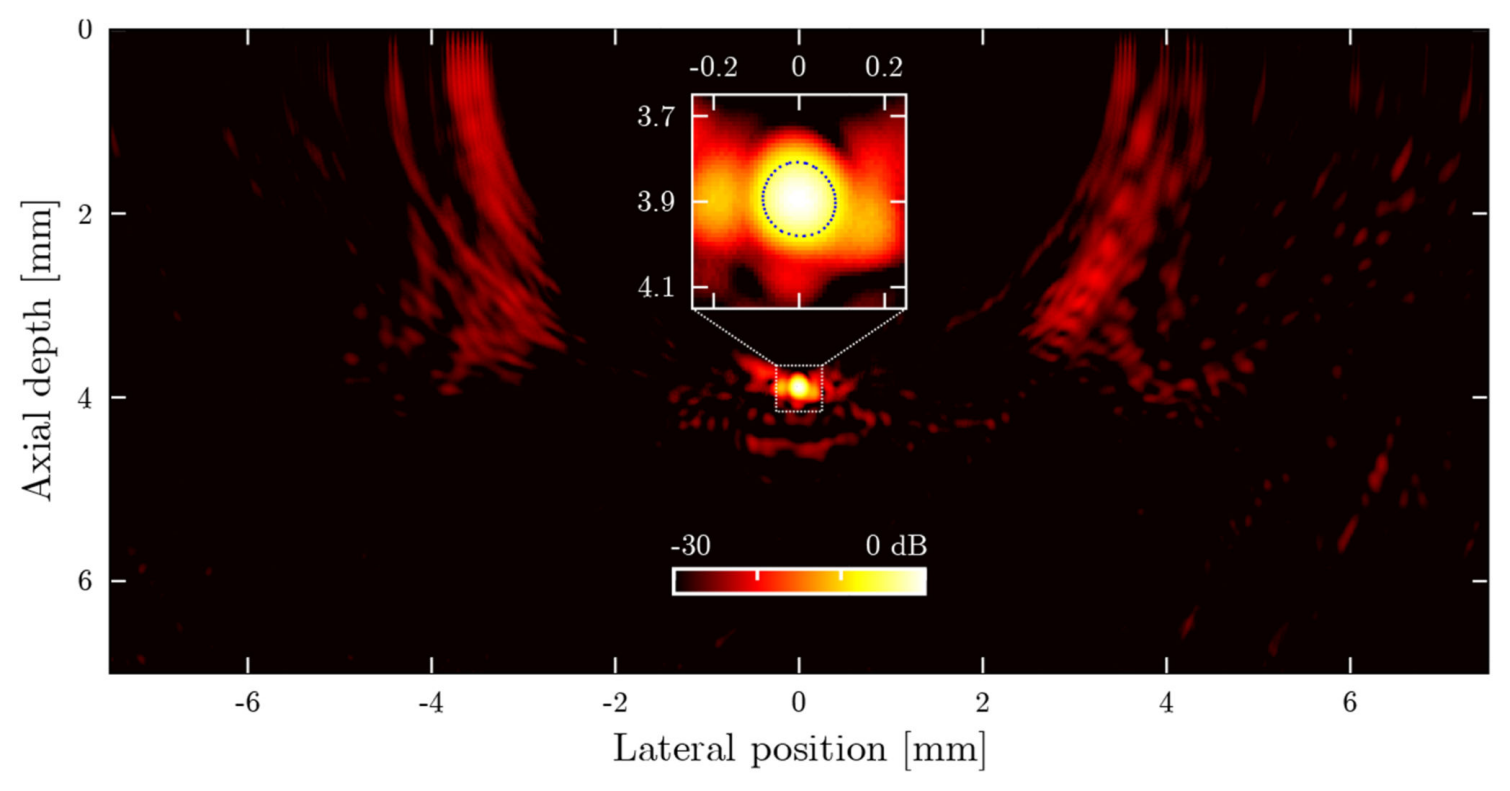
Point spread function of the all-optical ultrasound imaging system. All-optical ultrasound image of a tungsten wire (diameter: 27 μm; acting as a point target) placed centrally within the image at an axial depth of 3.9 mm. The insert shows a magnification of the image, and the –6 dB level corresponding to the spatial resolution of the imaging system is indicated by the dotted contour. The image is shown on a normalised logarithmic scale at a dynamic range of 30 dB.
